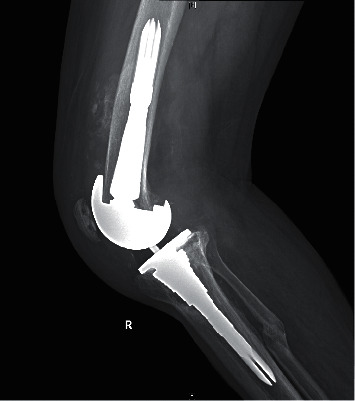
Lateral radiograph of the right knee showing the heterotopic ossification.
